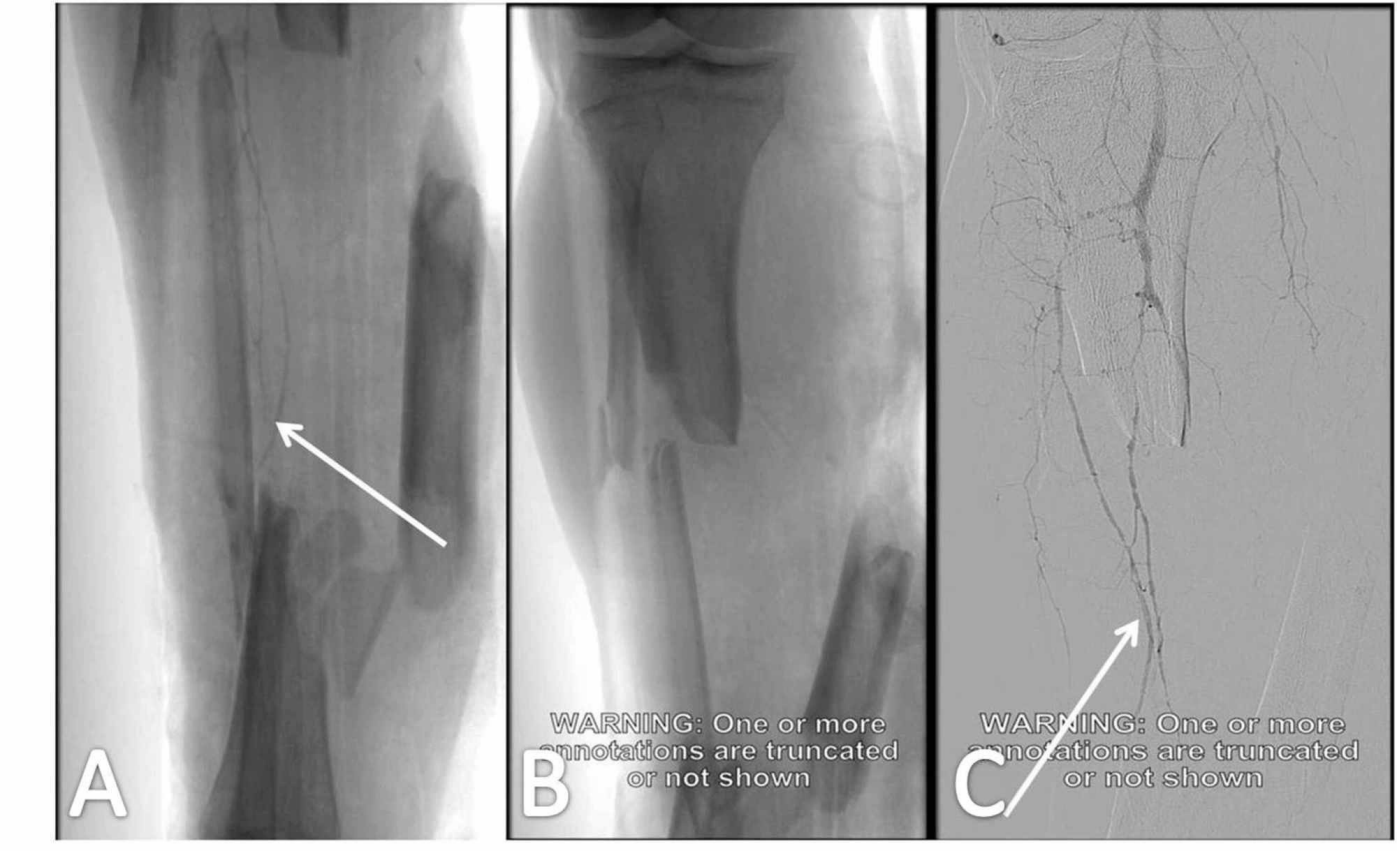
Vascular investigation with CT angiography Patent vascular network of the lower limb (white arrows in Figures A and C); comminuted and segmental fracture of the right tibial and fibular shaft (A, B) CT, computed tomography
